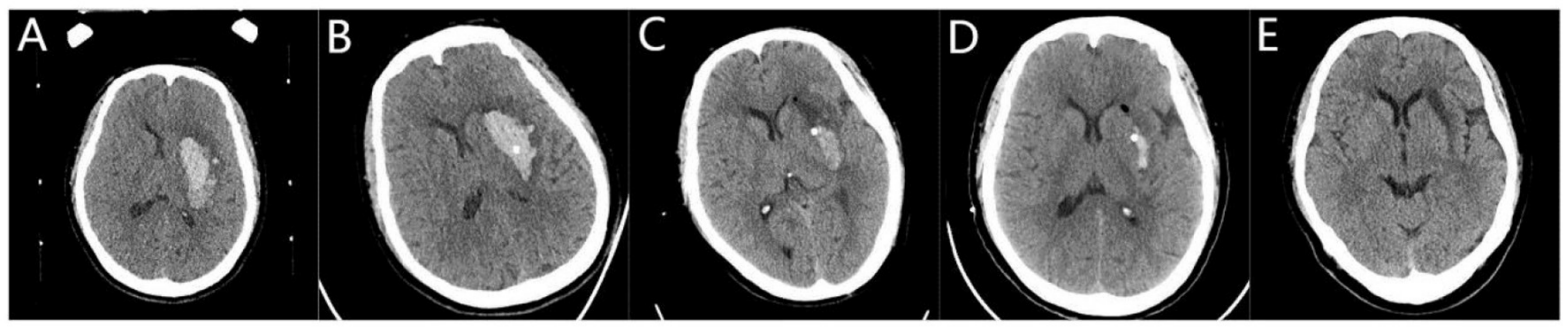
Stereotactic central catheterization for basal ganglia hemorrhage. (A) A preoperative head Computed Tomography (CT) showed that the volume of bleeding was ~26 ml. (B) A three-dimensional CT performed 6 h after surgery showed the drainage tube located in the center of the hematoma with a hematoma volume of ~17 ml. (C) Following the injection of urokinase, there was a small amount of residual hematoma on the third day after the operation, with a hematoma volume of 5 ml. (D) On the 7th day after surgery, the hematoma volume was 1.2 ml. (E) On the 20th day after surgery, the bleeding site softened.
